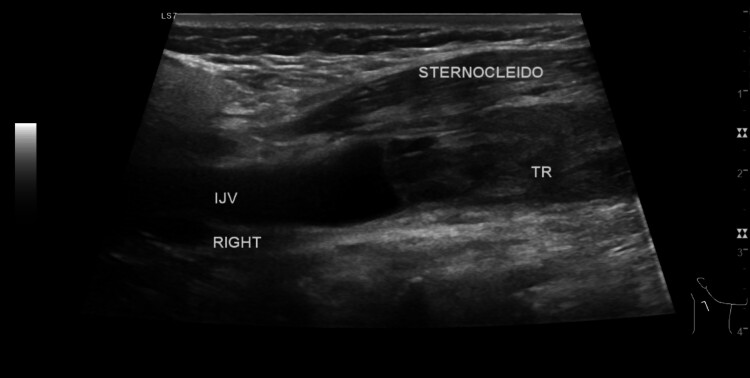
Neck ultrasound revealed intraluminal thrombus in the right internal jugular vein.
